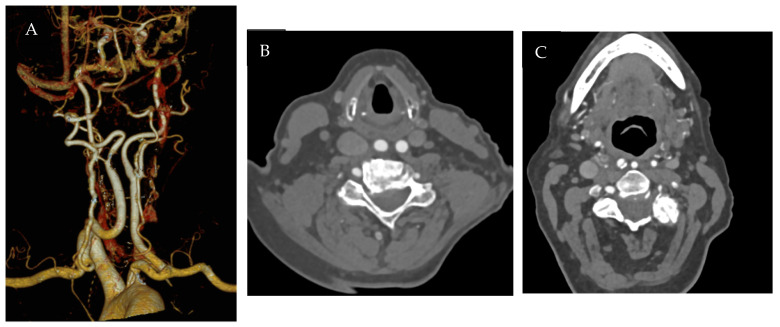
Computed tomography angiography images: (A) Retropharyngeal course of the internal carotid artery with 3D reconstruction. (B) Kissing carotids is the term used to identify an anatomical variant where two carotid arteries are located very close to each other on the midline. (C) Stenosis of the right internal carotid artery with a retropharyngeal course.
